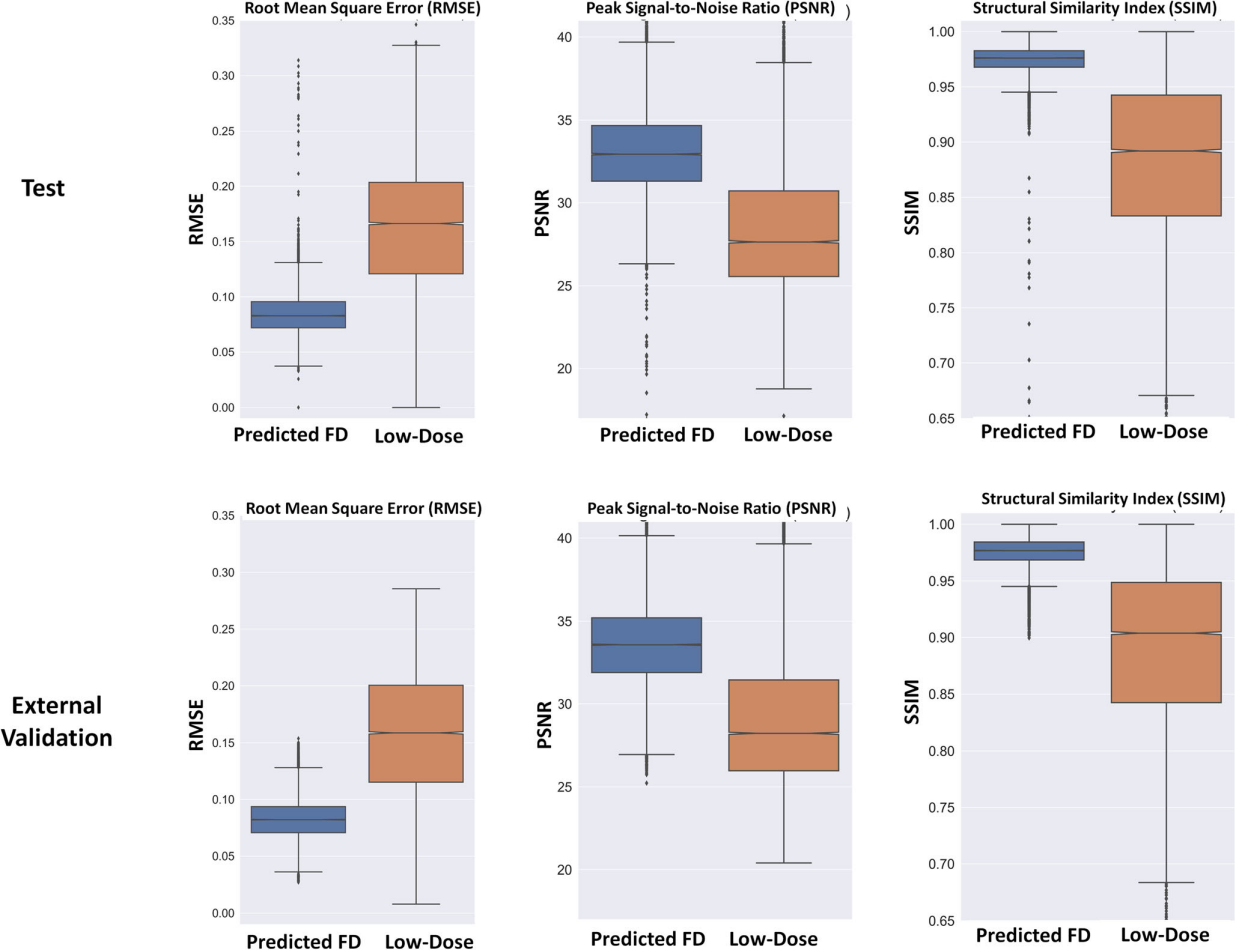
Mean and STD of peak signal-to-noise ratio (PSNR), structural similarity index (SSIM), and root mean square error (RMSE) for the predicted and ultra-low-dose CT images in the test and external validation sets
